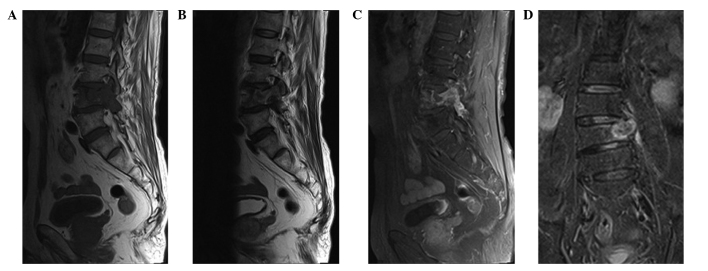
Magentic resonance imaging detected a cystic, destructive lesion in the L4 vertebra which exhibited a soft tissue component. (A) On T1-weighted sagittal image of the L4 vertebra, an intraosseous lesion with low signal intensity was observed and (B) on T2-weighted image, the lesion was slightly hyperintense. (C) Using short TI inversion-recovery imaging, the lesion exhibited high intensities. (D) On contrast-enhanced T1-weighted image, the lesion was homogeneously enhanced and was found to be compressing the adjacent spinal cord. L4, fourth lumbar.
